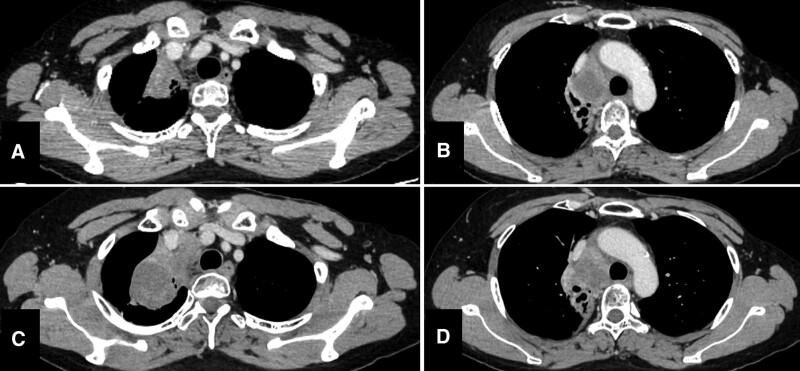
Contrast enhanced computed tomography of the tumor during entrectinib therapy. After 1 mo of entrectinib at 600 mg/day had been administered, CT showed stable disease (A, B). However, the tumor started to grow (C, D) after 5 mo of medication. CT = computed tomography.
